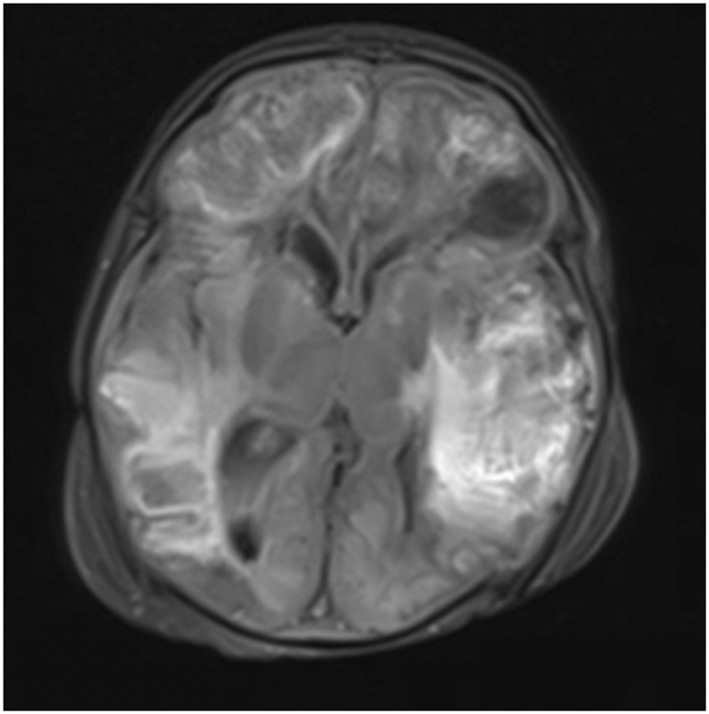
MRI brain with contrast flair axial view revealing significant progression of disease with extensive multifocal bilateral intraparenchymal hemorrhage.
